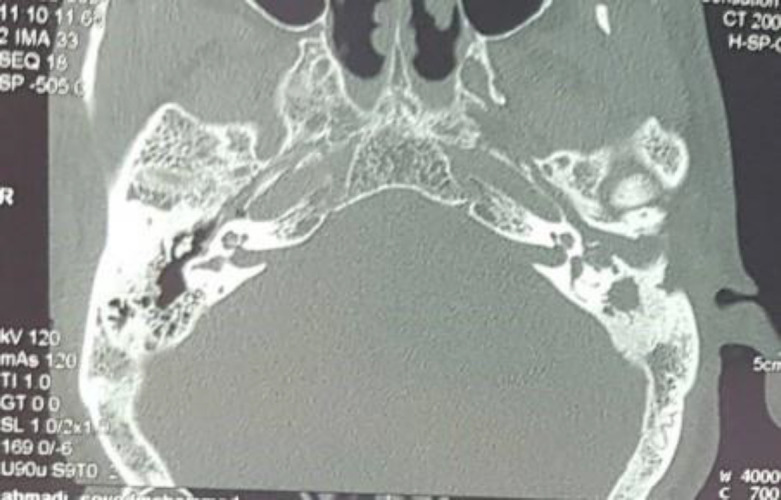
Coronal temporal CT scan shows sclerotic left mastoid due to chronic matoiditis and soft tissue density in mastoid middle ear and external auditary
